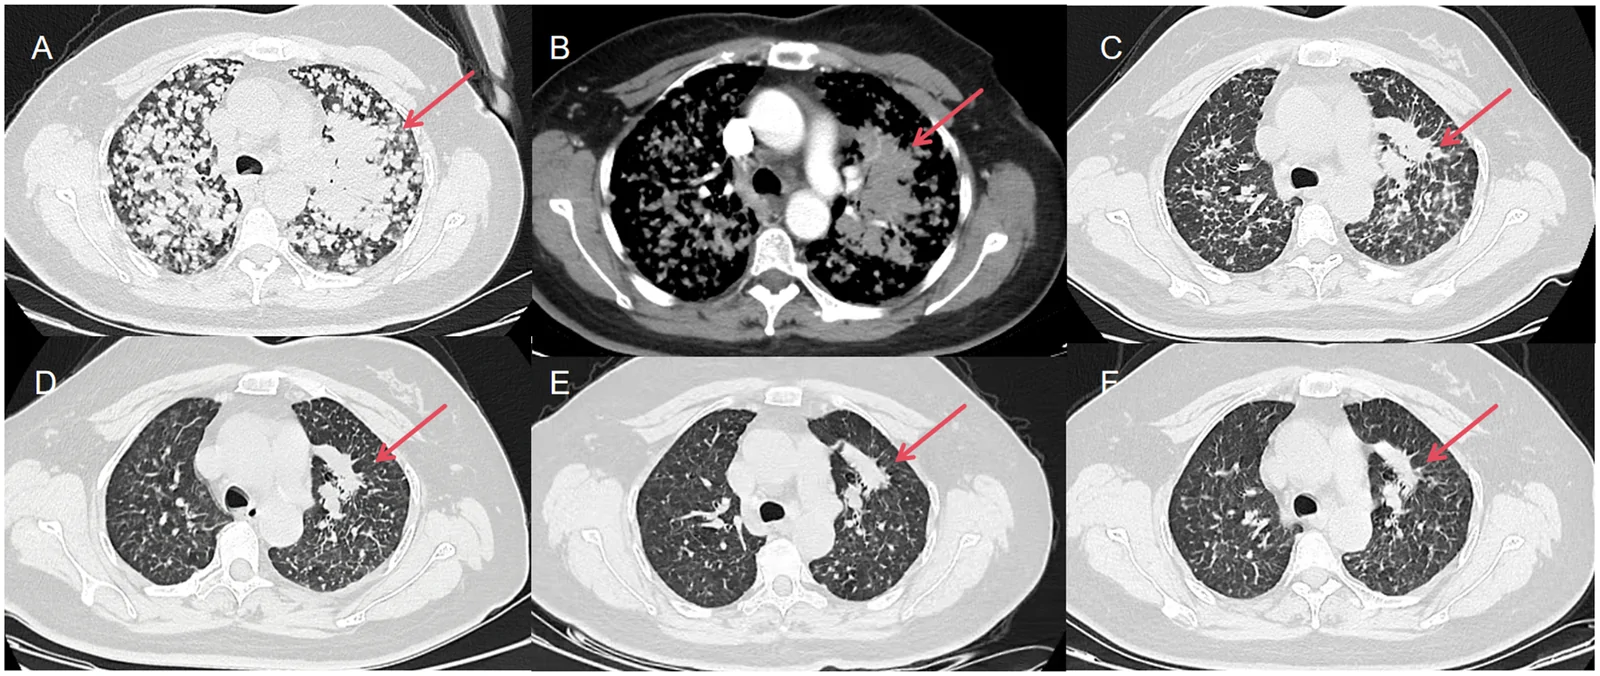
Chronological chest CT evaluation before and after aumolertinib treatment. (A, B) Baseline evaluation: (A) Lung window image showing diffuse bilateral solid nodules with a dominant lesion in the left upper lung (red arrows, 57 mm); (B) Contrast-enhanced mediastinal window image demonstrating mild enhancement of the dominant lesion and the absence of significant mediastinal or hilar lymphadenopathy. (C–F) Follow-up lung windows demonstrating therapeutic response over time: (C) 1st Assessment (PR): Dominant lesion: 33 mm (D) 2nd Assessment (PR): Dominant lesion: 29 mm; lymphangitic improvement; (E) 3rd Assessment (PR): Dominant lesion: 28 mm (F) 4th Assessment (PR): Dominant lesion: 28 mm.
